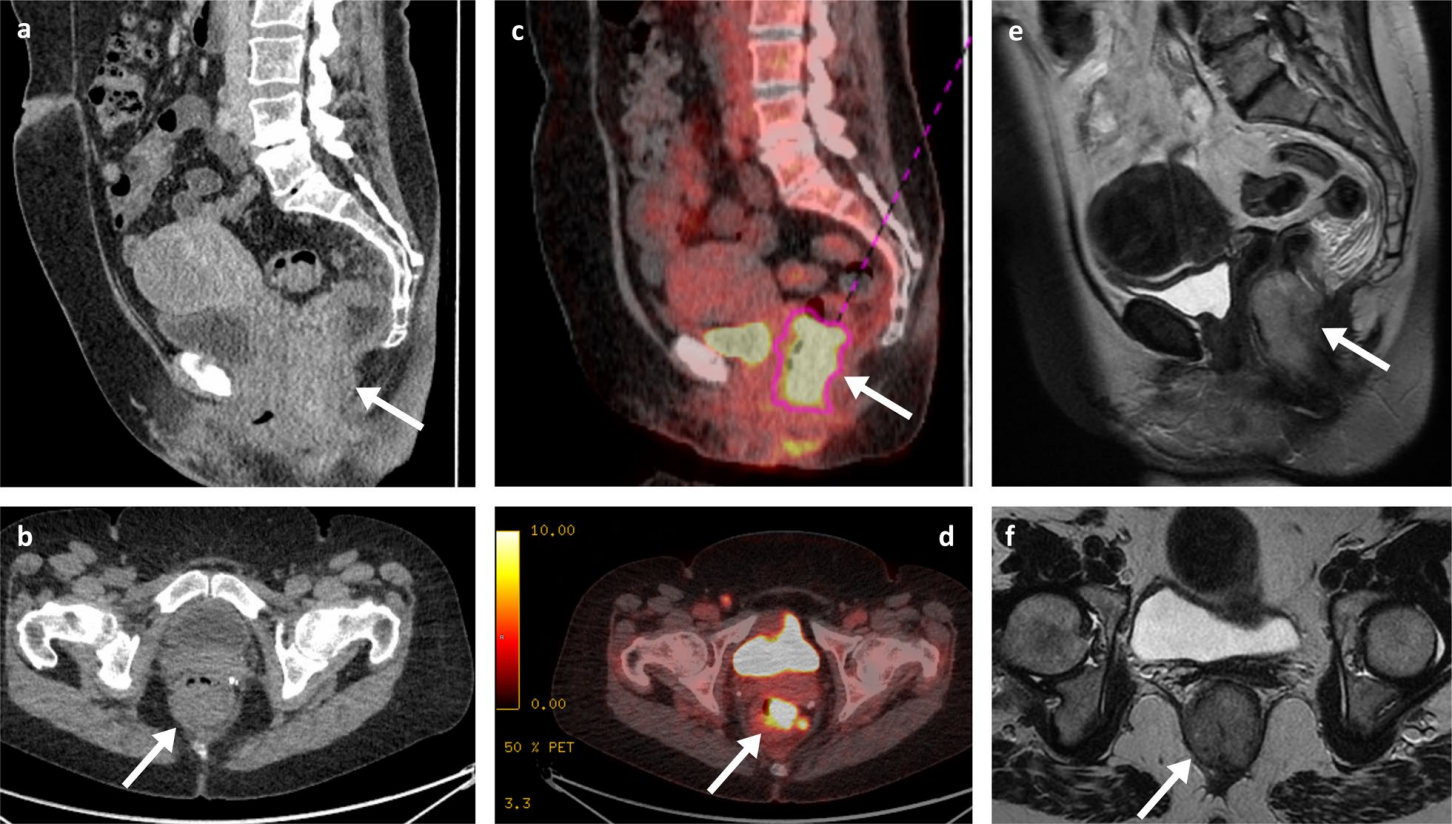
Portal phase contrast-enhanced CT sagittal (a) and axial (b), PET-CT sagittal (c) and axial (d), and MRI T2WI sagittal (e) and T2WI axial (f) of a 57-year-old female patient with an anorectal metastatic melanoma (arrows) with a craniocaudal length of 5 cm, situated 1.3 cm from the anal verge with involvement of the right internal sphincter complex
